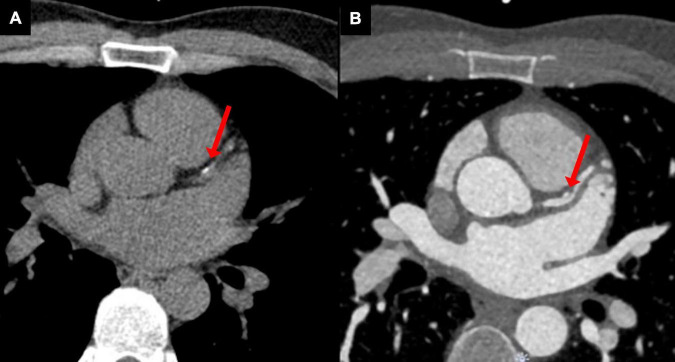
Young patient treated with radiotherapy for Hodgkin lymphoma in childhood with premature atherosclerosis. (A) A non-contrast computed tomography scan, used for the assessment of coronary artery calcium score, showed calcinations in the proximal left anterior descending coronary artery (red arrow). (B) Coronary computed tomography angiography revealed a non-obstructive vulnerable plaque (red arrow).
